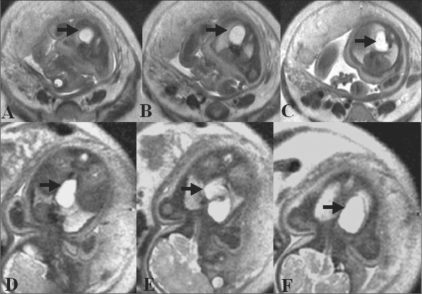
Antenatal MRI: Axial (A–C) and coronal (D–F) sections show the thoracoabdominal extent of the posterior mediastinal cyst (arrows). The lesion is in the right paravertebral region in the upper thorax and in the prevertebral region in the lower thorax
